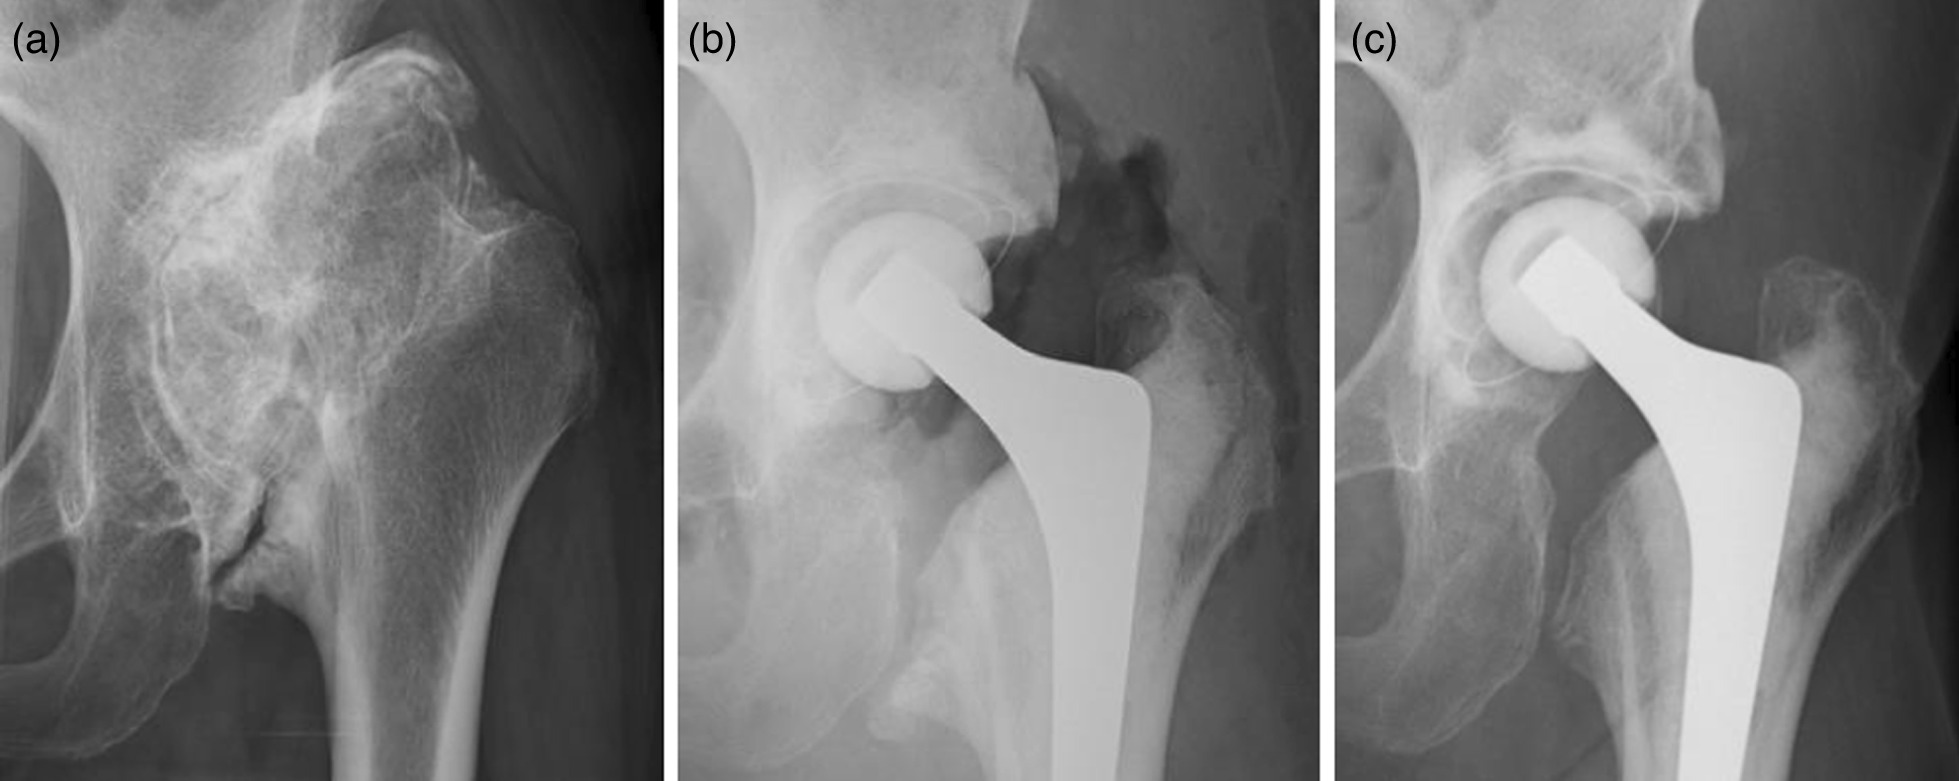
AP radiographs of the patient who underwent cTHA via this method. a Preoperative AP radiograph. b Postoperative AP radiograph. Two Superfixorb screws, 35 mm and 45 mm, were used. c AP radiograph at 2 years of follow-up
